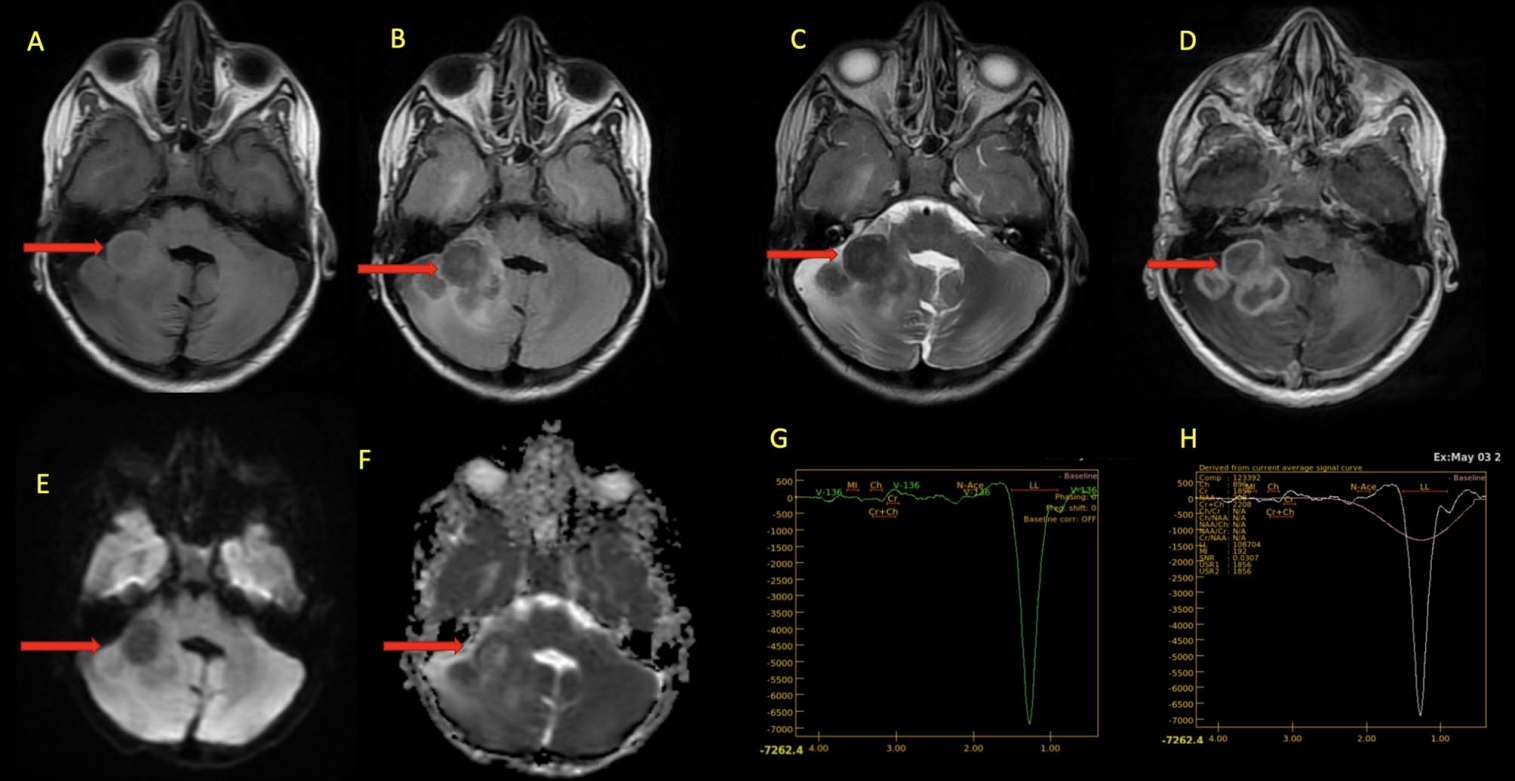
MRI brain. Axial images in T1W (A), FLAIR (B), T2W (C), post contrast T1 fat suppressed (D), DWI (E) and ADC (F) sequences showing tuberculomas in right cerebellar region (red arrow) appearing iso-hypointense on T1, T2 and FLAIR images; with postcontrast peripheral rim enhancement, no restricted diffusion with mild surrounding oedema and mass effect mildly compressing fourth ventricle and obliterating right CP angle cistern. MRS images (G & H) revealed high lipid lactate peak, reduced or Absent NAA, Choline, and Creatine
